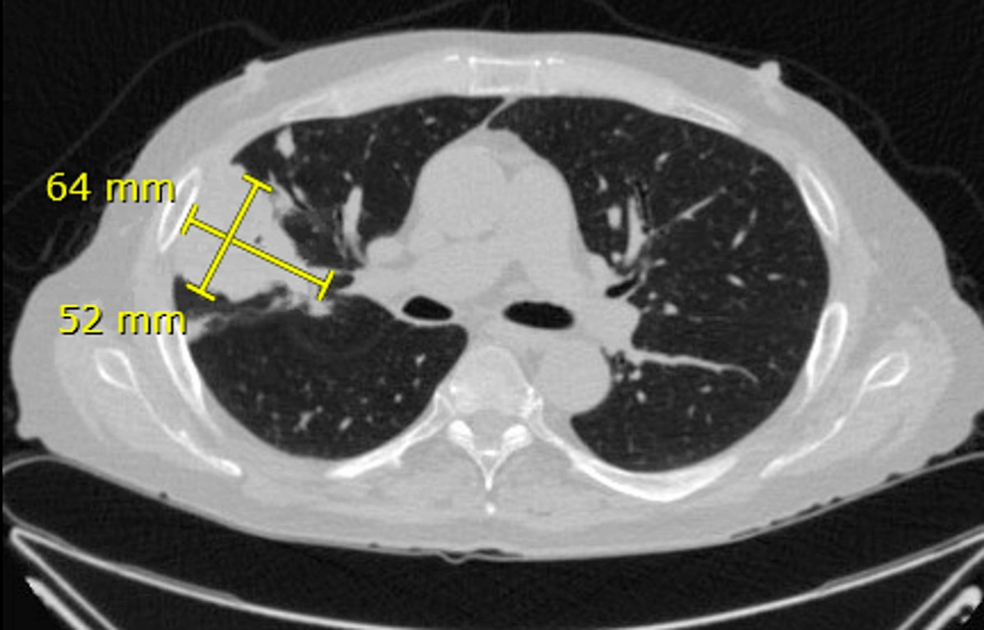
Axial view of repeat CT chest two months after initial CT, re-demonstrating dominant mass in the right upper lobe, measuring up to 6.4 x 5.2 cm.
